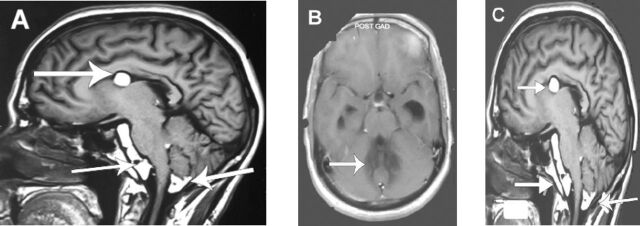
Pre-op MRI with contrast a) Pre–op (T1 MRI)- Fat in the prepontine, cerebellomedullary cistern and fourth ventricle with hydrocephalus, b) Marked area shows intensity changes in vermis (non enhancement on contrast) possible site of dermoid cyst rupture c) post-op MRI (T1) fat in the same regions with ventricle decompression. Also fat visualised in the lateral ventricle.
